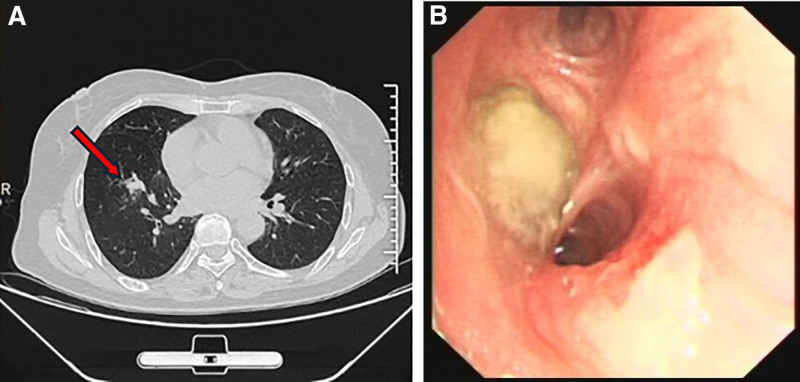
(A) CT scan revealed a cast-like high-density shadow in the right lower lobe of the lung. (B) Bronchoscopy revealed mucosal swelling and thick white mucous plugs in the right lower bronchus, which could be partially aspirated. CT = computed tomography.
